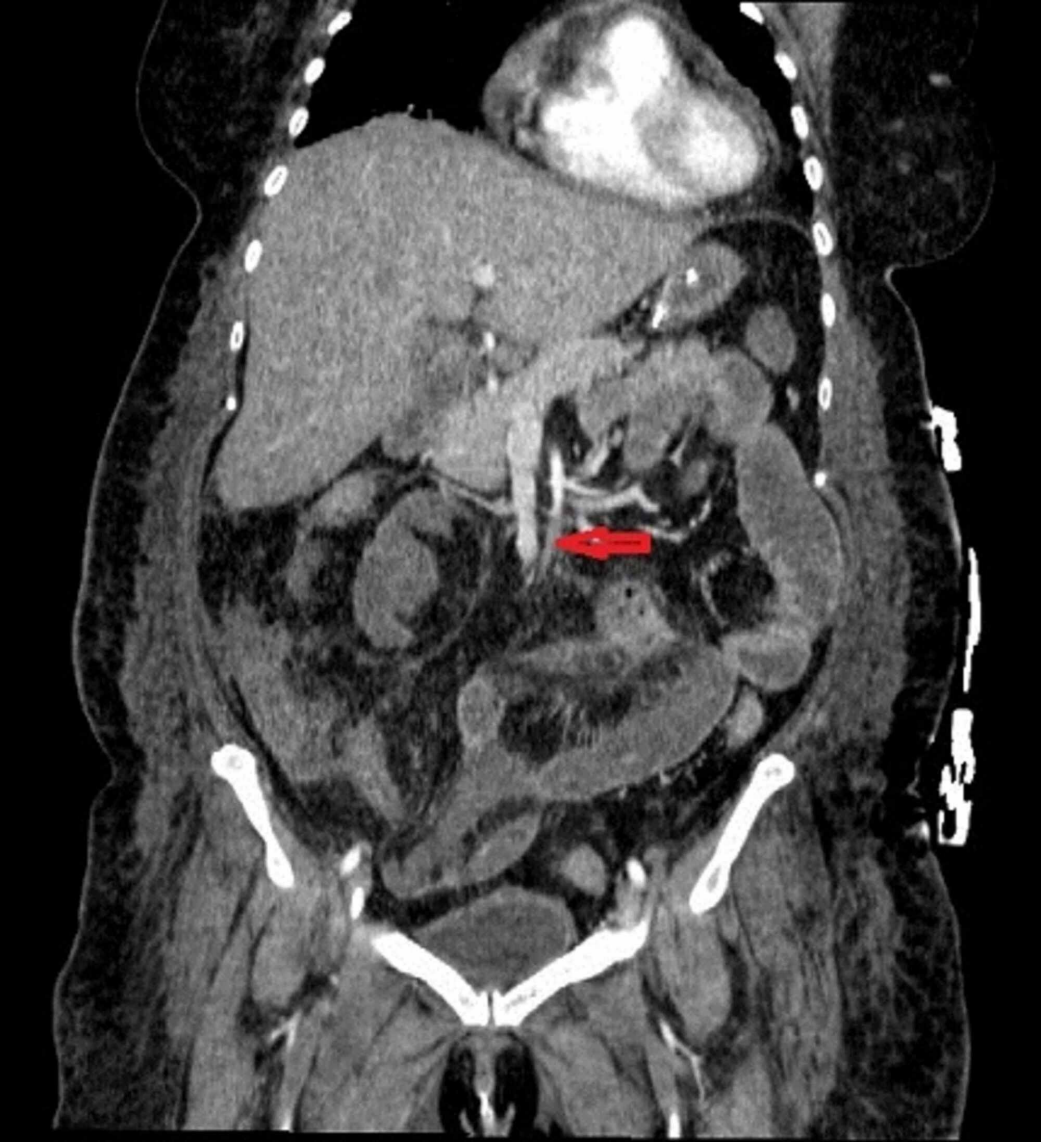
CT scan coronal view: upper mesenteric artery thrombosis (arrow)
